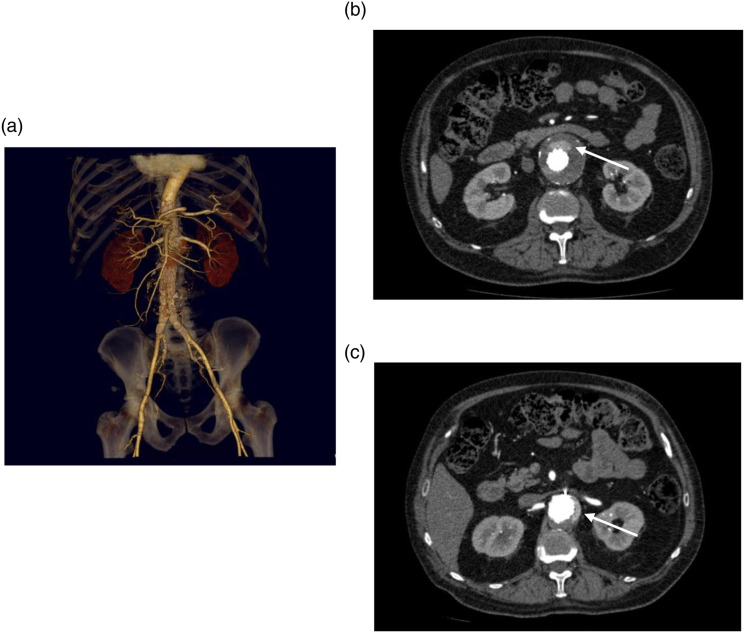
(a) Reconstruction of conventional CTA for 4 fenestrated endograft. (b and c) demonstrate representative axial images of the CTA with endoleak of unclear origin within the anterior and left aneurysm sac (white arrows).
